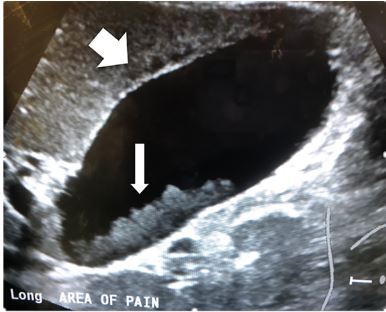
Formal ultrasonographic image showing a distended gallbladder in long axis with scant pericholecystic fluid (thick arrow) and sludge (thin arrow).
